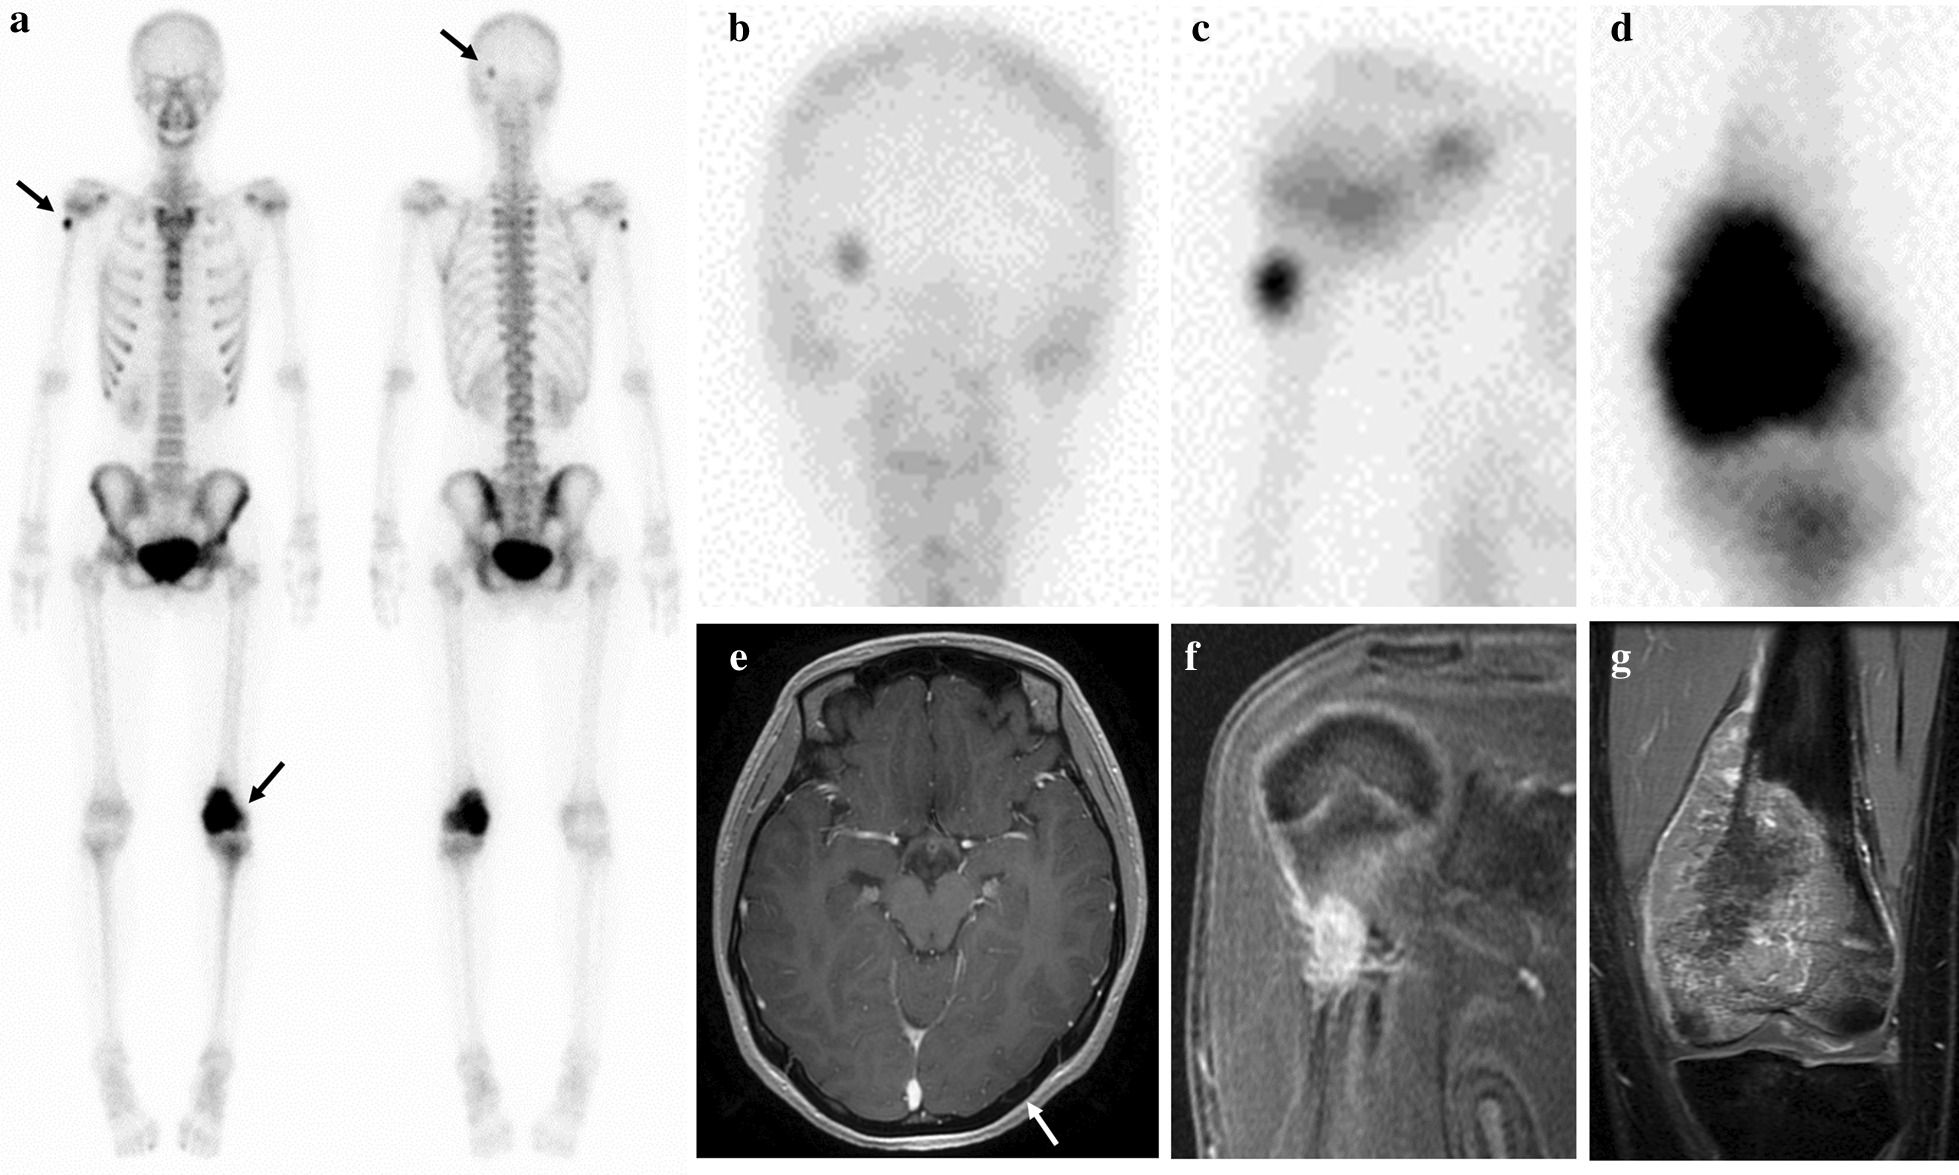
Systemic bone scan and MRI findings before neoadjuvant chemotherapy. a–c Bone scan revealed uptake for the following three location (a); left occipital bone (b), right proximal humerus (c), and left distal femur (d). e The left occipital lesion on axial enhanced MRI, and a white arrow shows the intracortical lesion. f The right proximal humeral lesion on coronal enhanced MRI. g The left distal femoral lesion on coronal enhanced MRI
